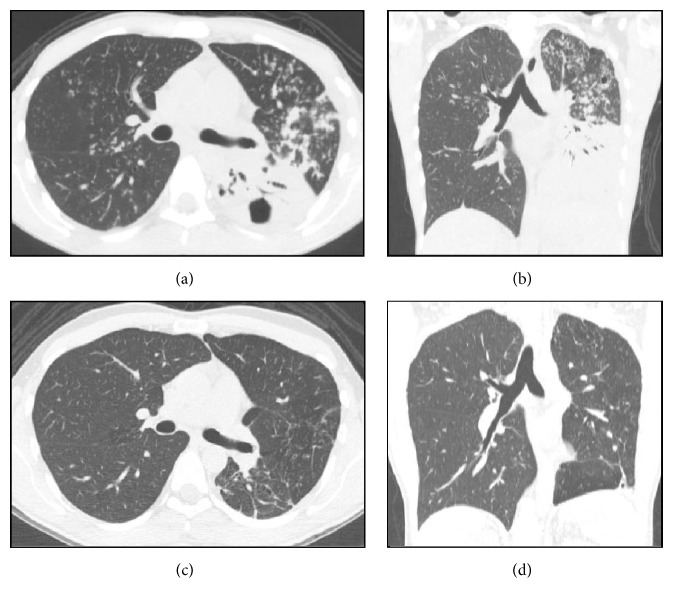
Computed tomography findings in TB. Axial view (a) and coronal reformatting (b) of TB before treatment, recorded in parenchymal window, indicating extensive consolidations intermingled with air bronchograms in the left lower lobe, associated with cavitary lesions and draining bronchus, confluent opacities, and bronchogenic dissemination characterized by airway nodules configuring the tree-in-bud pattern observed in the left upper lobe and right lung. Additionally, note the airway changes represented by increased wall thickness and bronchial dilation in the left upper lobe. (c) and (d) show the structural changes observed after treatment, characterized by volumetric reduction of the left lung, architectural distortions, thick bands of fibrous aspect on the left base, and bronchial changes in the left upper lobe.
